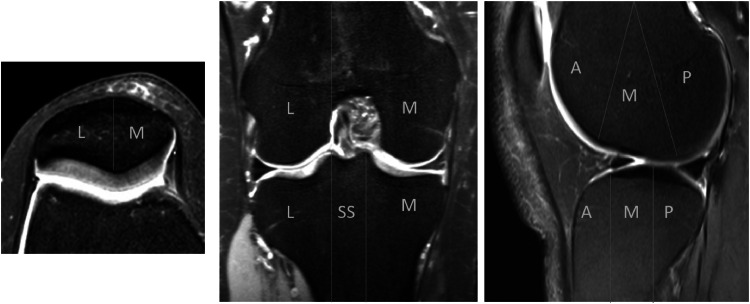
Retrospective study to evaluate the impact of AI assistance on the diagnostic accuracy of radiologists for detecting bone marrow edema in knee MRI: The 15 subregions of the knee bones, defined according to MOAKS classification criteria. In the leftmost image, the patella is divided into two subregions. In the middle and right images, both the femur and tibia are each divided into three subregions for the medial and lateral compartments.
